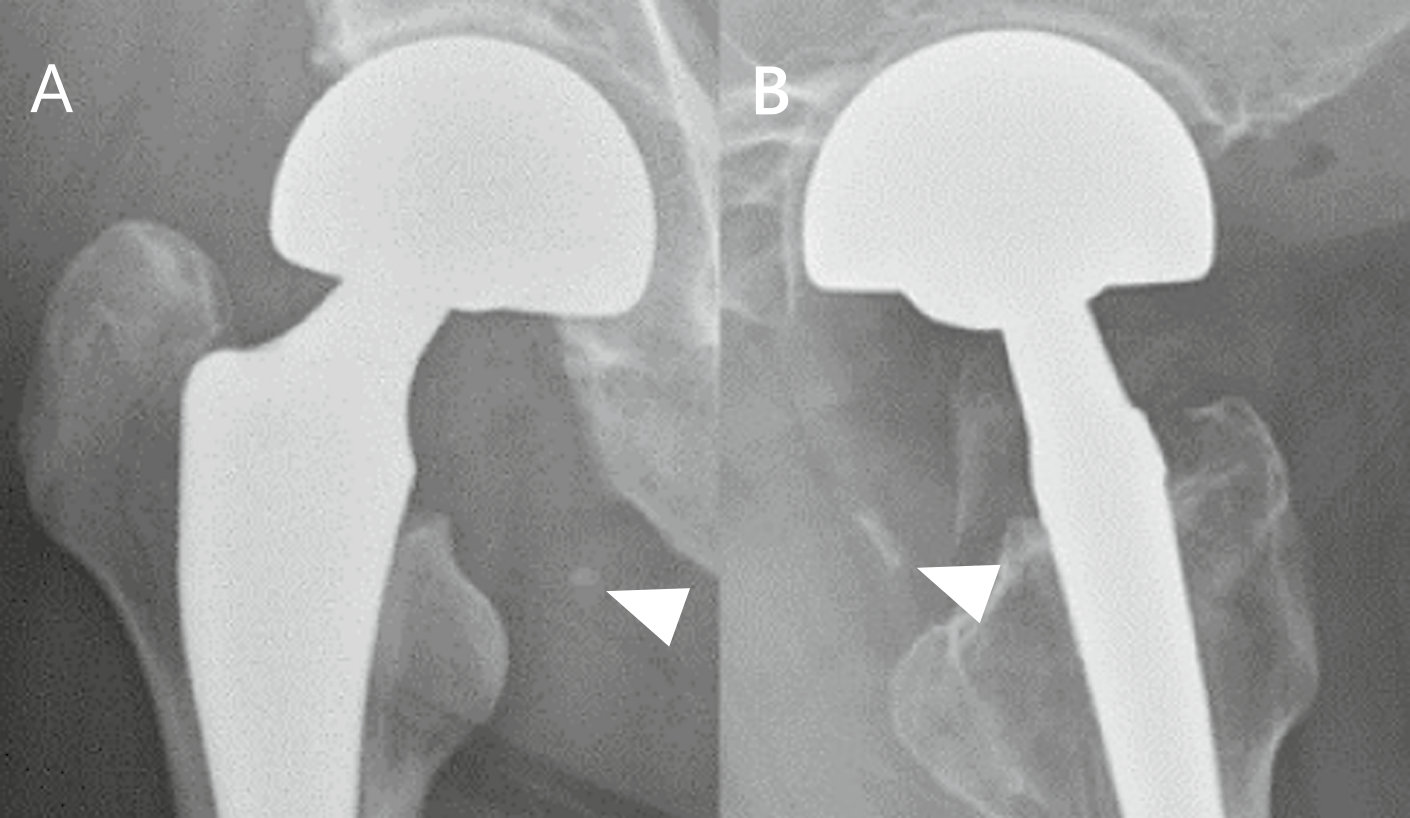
Free bone fragment immediately after surgery. A Anteroposterior radiograph. Arrowhead shows free bone fragment. B Lateral radiograph. Arrowhead shows free bone fragment
